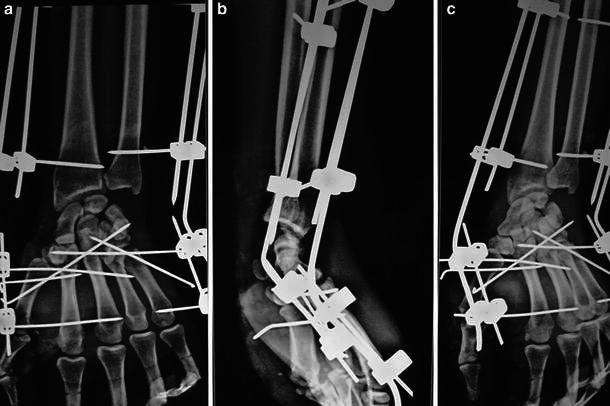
Immediate postoperative radiographs (anteroposterior, lateral, and oblique views) showing the maintained reduction of the second, third, and fourth carpometacarpal joints stabilized with Kirschner wires and the mini external fixator
